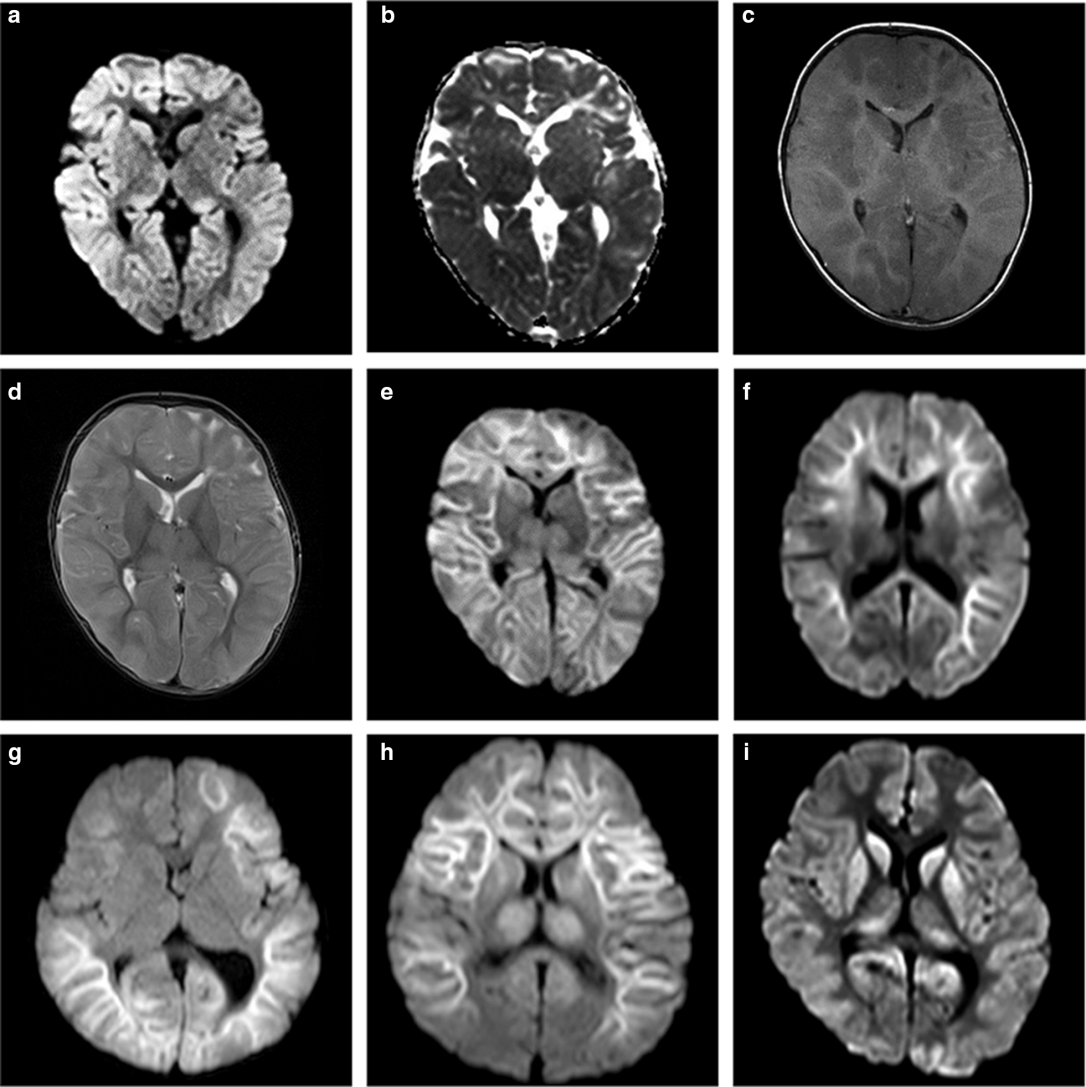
MRI findings. a, b MRI findings in patient 1 at the onset of acute encephalopathy (AE). Reduced water diffusion was evident over the entire cerebral cortex and the posterior parts of the thalami (a diffusion-weighted images; b apparent diffusion coefficient map). c–e MRI of patient 1 performed 4 days after AE onset. Marked brain edema with narrowing of the lateral ventricles was seen on T1- (c) and T2-weighted (d) images. Diffusion-weighted images exhibited reduced water diffusion, predominantly in the subcortical white matter (e). f MRI of patient 3 performed 7 days after AE onset. Diffusion-weighted images revealed reduced water diffusion in the subcortical white matter and corpus callosum. g MRI of patient 4 performed 3 days after AE onset. Reduced water diffusion was evident in the subcortical white matter of the left frontal and bilateral temporo–parieto–occipital areas. h MRI of patient 6 performed 5 days after AE onset. Reduced water diffusion was evident in the bilateral thalami and subcortical white matter, except for the bilateral occipital areas. i MRI of patient 2 performed 17 days after AE onset. Diffusion-weighted images revealed reduced water diffusion in the caudate nuclei, basal ganglia, and the pulvinar thalami
